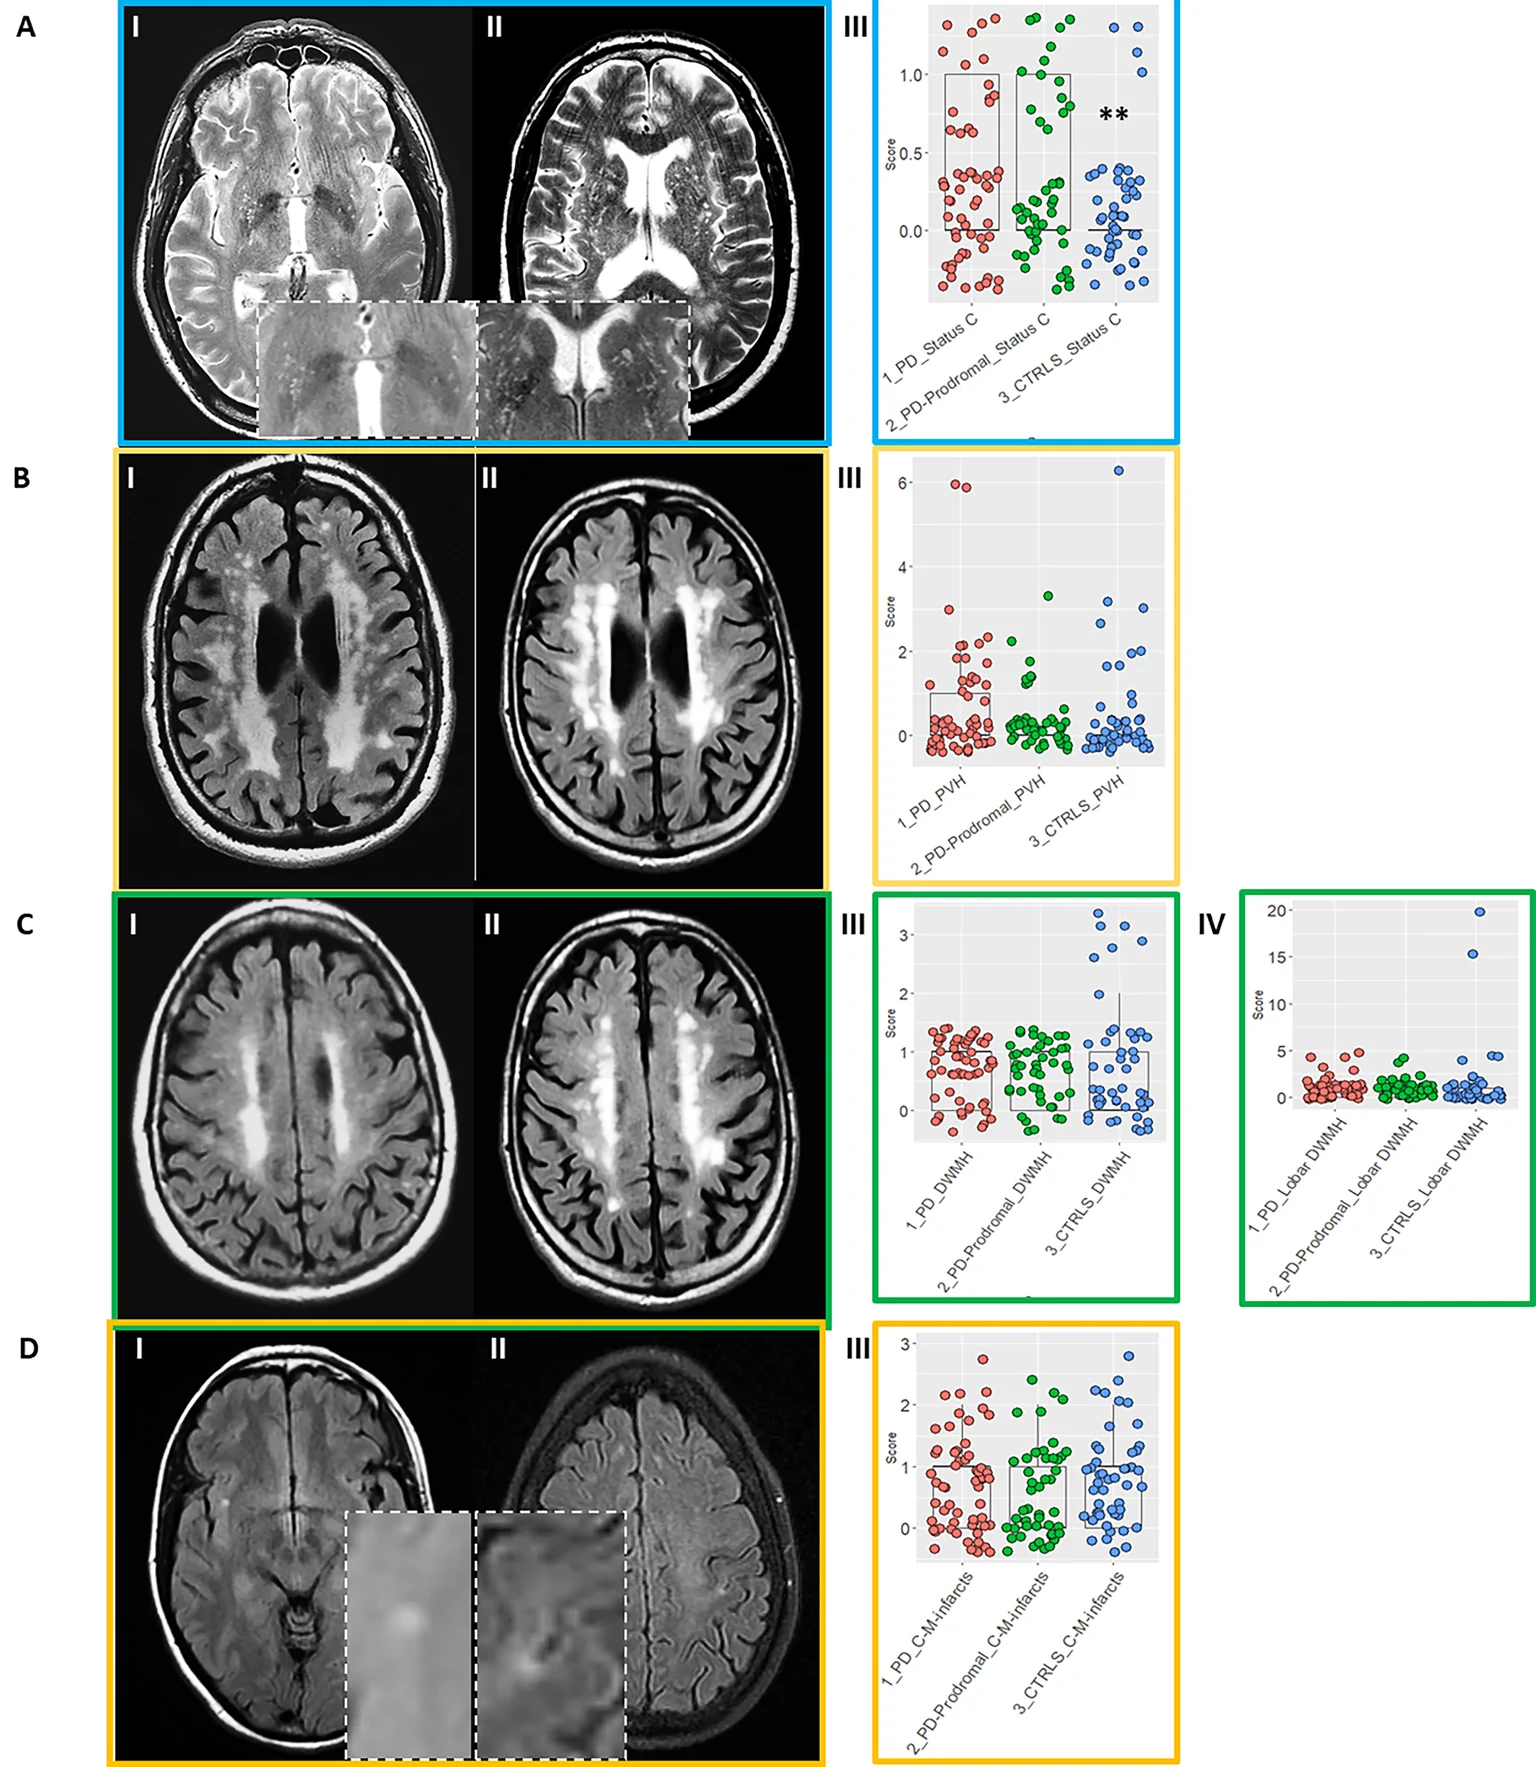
A-D. Axial T2-FLAIR MRI scans displaying enlarged perivascular spaces, periventricular white matter hyperintensities, deep white matter hyperintensities, cortical microinfarcts and corresponding box-dot-plots of PD and PD prodromal patients and controls. A. Enlarged perivascular spaces. I Control, 74 years old, homozygous for *APOE* ε3, with a very mild motor impairment (MDS-UPDRS-III 3), and subtle cognitive impairment (MoCA 25). II PD patient, 71 years old, not carrying any rare known pathogenic mutation in *LRRK2*, *GBA* and *SNCA*, heterozygous for *APOE* ε4 (ε3/ε4), presenting a severe motor impairment (MDS-UPDRS-III 31) and mild cognitive impairment (MoCA 20). III Box-dot plot displaying a significant enrichment of basal ganglia enlarged perivascular spaces in PD and PD prodromal patients (p-value = 2.64e-03, Bonferroni-corrected) compared to controls. ^^ p-value < 0,005. B
